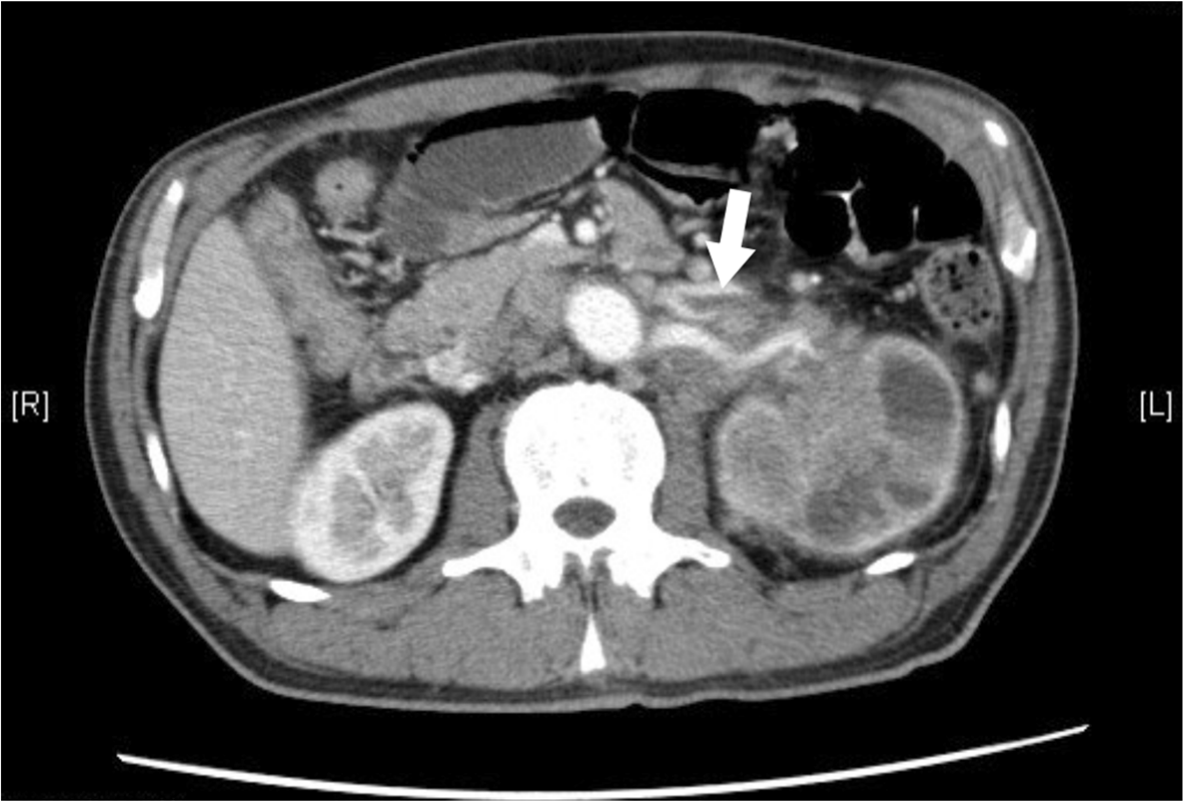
Contrast-enhanced computed tomography showed that tumor invading the left renal vein (arrow)
